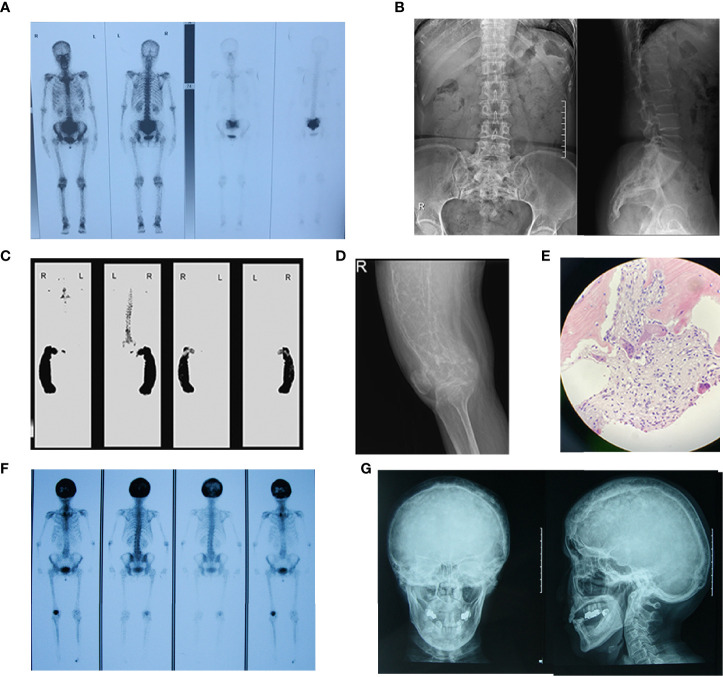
Bone scintigraphy and X-ray images of patients with PDB. (A) Bone scintigraphy image depicted with high 99^m^Tc-MDP uptake in sacral vertebrae. (B) The frontal and lateral pelvic radiograph showed heterogeneous sclerosis density involving sacral vertebrae. (C) The bone scan showed enlargement and bending in a “knife arc” pattern of the left femur, accompanied by high 99^m^Tc-MDP uptake in the left femur. (D) The radiograph of the left femur revealed structural changes with significant bowing, cortical destruction and dilation. The trabecular bones were coarse and arrayed irregularly, showing a “towel gourd sac-like” appearance and loss of the normally clear distinction between the cancellous bone and cortical bone. (E) Biopsy of the left femoral mass revealed osteoclastic giant cells and bone resorption with fibrous tissue hyperplasia. Patient 12 F Bone scintigraphy image depicted with high 99^m^Tc-MDP uptake in the skull. (G) The plain skull film showed craniocerebral thickening with the skull plate barrier disappearing. The cranial suture could not be clearly displayed with the mixture of high-density and low-density areas, and the sclerotic foci showed a dense cotton spherical shadow.
